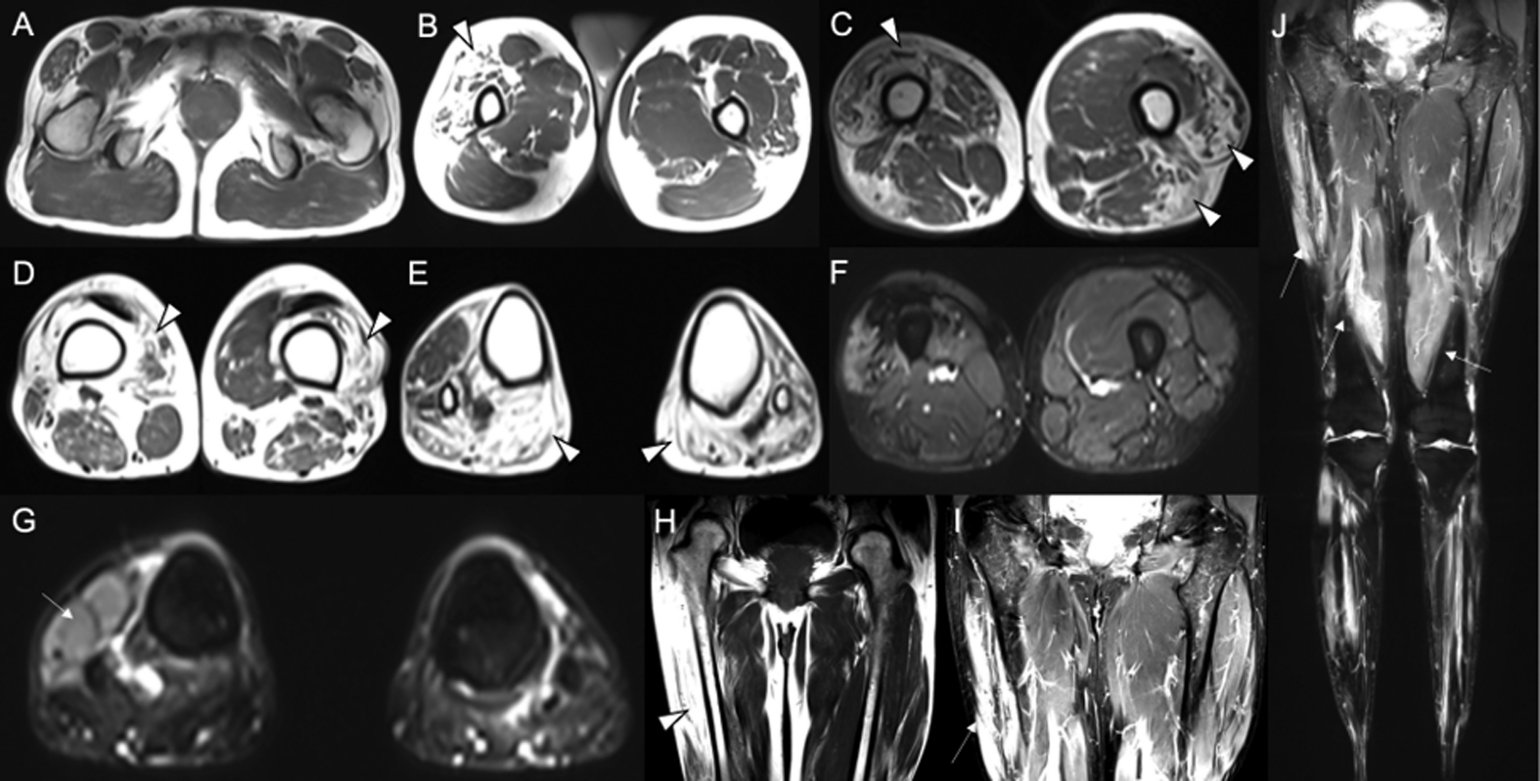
Muscle MRI studies. Axial (
